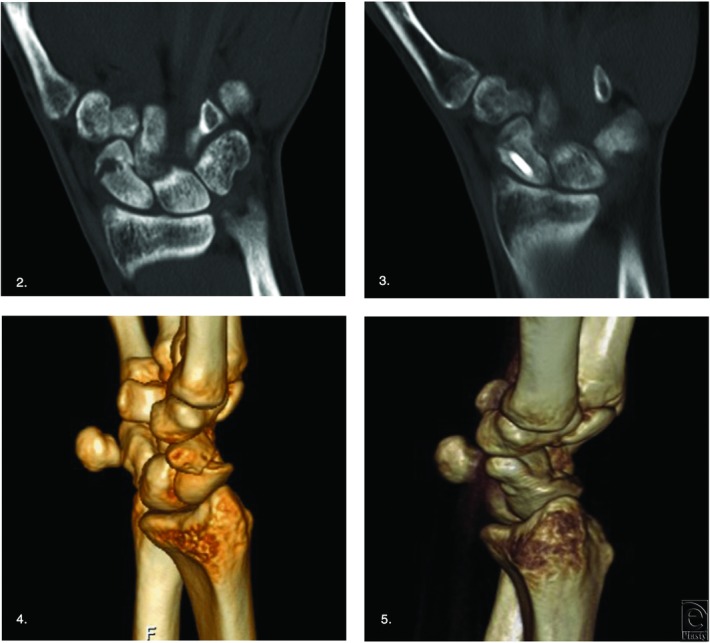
Computed tomographic and 3-dimensional computed tomographic scans of a scaphoid nonunion with corrected humpback deformity (pre- and postoperative).
